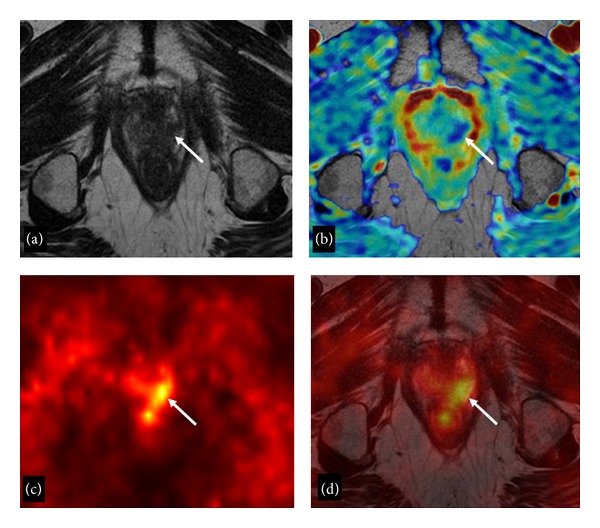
75-year-old man with biopsy-proven local PC recurrence after EBRT. T2-weighted axial image (a) demonstrates a hypointense area (arrow) in the posterior left lateral aspect of the prostate apex. The ADC map (b) demonstrates that the hypointense nodular area has lower ADC values than the surrounding parenchyma (arrow). On ^18^F-Choline-PET axial scan (c) a well-defined PET-positive focus is appreciable (arrow). Fused ^18^F-Choline-PET/MRI image (d) shows exact correspondence between PET-positive focus and MRI finding (arrow).
